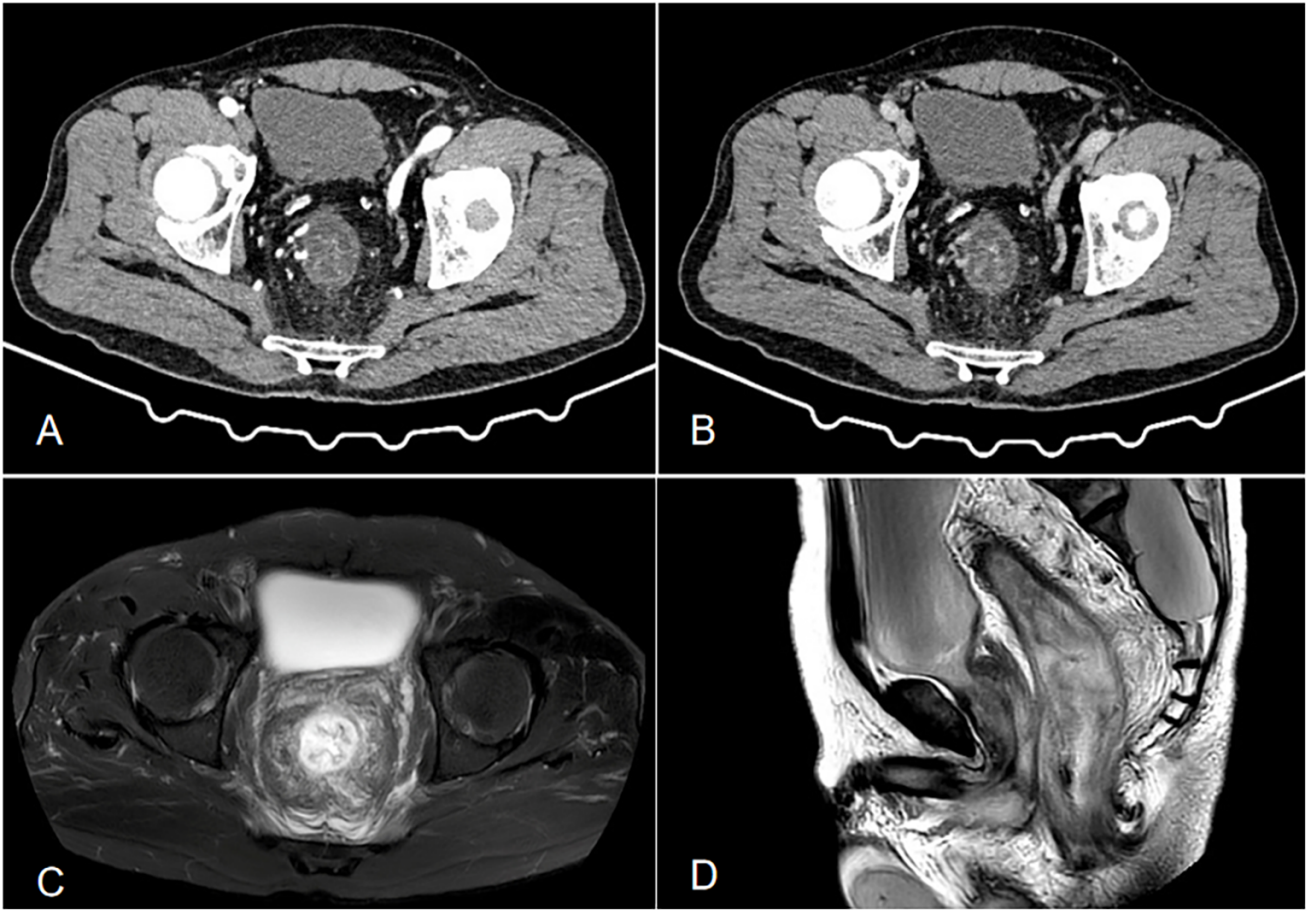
(A, B) Contrast-enhanced CT shows local wall thickening and heterogeneous enhancement of the sigmoid colon, with increased and thickened surrounding blood vessels, dilation and effusion of the proximal intestinal lumen, thickening of the distal rectosigmoid colon wall showing a layered change, and layered enhancement after enhancement. Its feeding artery is thickened, with exudation and fascia thickening seen around(a is the venous phase, and b is the arterial phase). (C, D) Enhanced pelvic MRI demonstrated segmental wall thickening of the rectum and sigmoid colon with marked blurring in the perirectal fat plane. (c is T2-weighted fat-suppressed sequence, and is Sagittal T2WI sequence).
